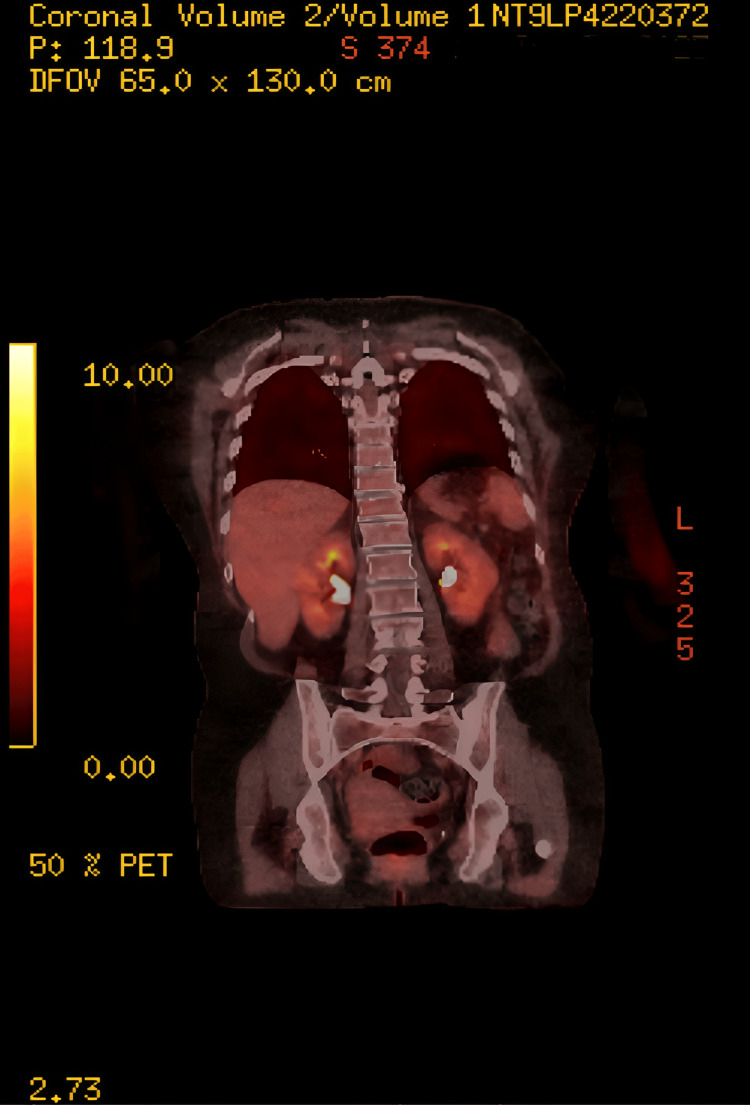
Nuclear medicine whole-body FDG PET/CT revealed a highly avid left adrenal mass. Note the diffuse uptake in the contralateral gland, likely representing an adrenal reaction to hormonal hypersecretion from the nodule FDG PET: fluorodeoxyglucose positron emission tomography; CT: computed tomography
